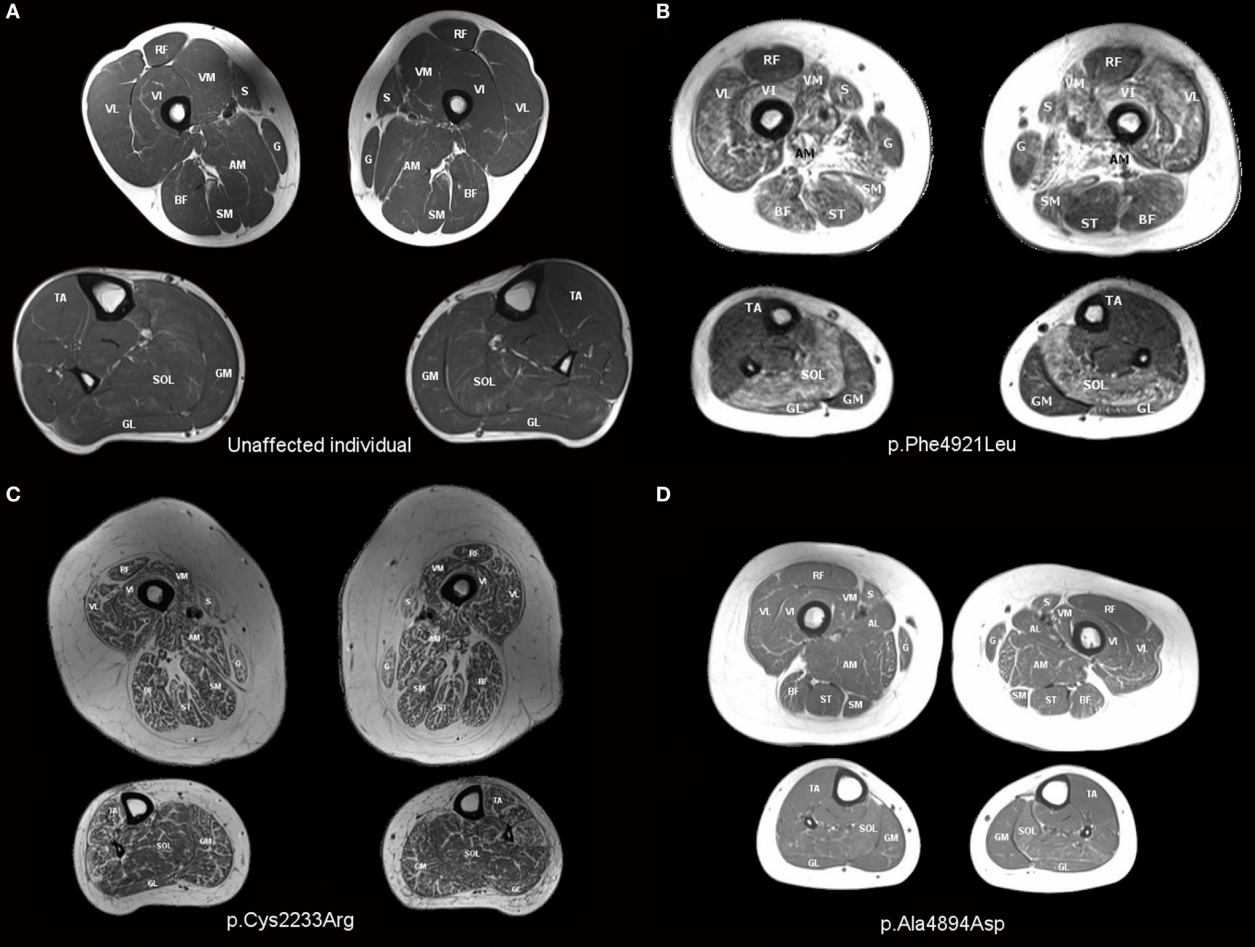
T1-weighted transverse MR images of the thigh and calf of an unaffected individual (A) and those with *RYR1*-RM (B–D). (B) Case 1: selective muscle involvement typical of *RYR1*-RM with sparing of rectus femoris, gracilis, and tibialis anterior, (C) Case 4: global muscle involvement, and (D) Case 3: asymmetrical muscle involvement with the individual’s right side affected to a lesser extent than the left. This is particularly evident in biceps femoris and vastus lateralis.
